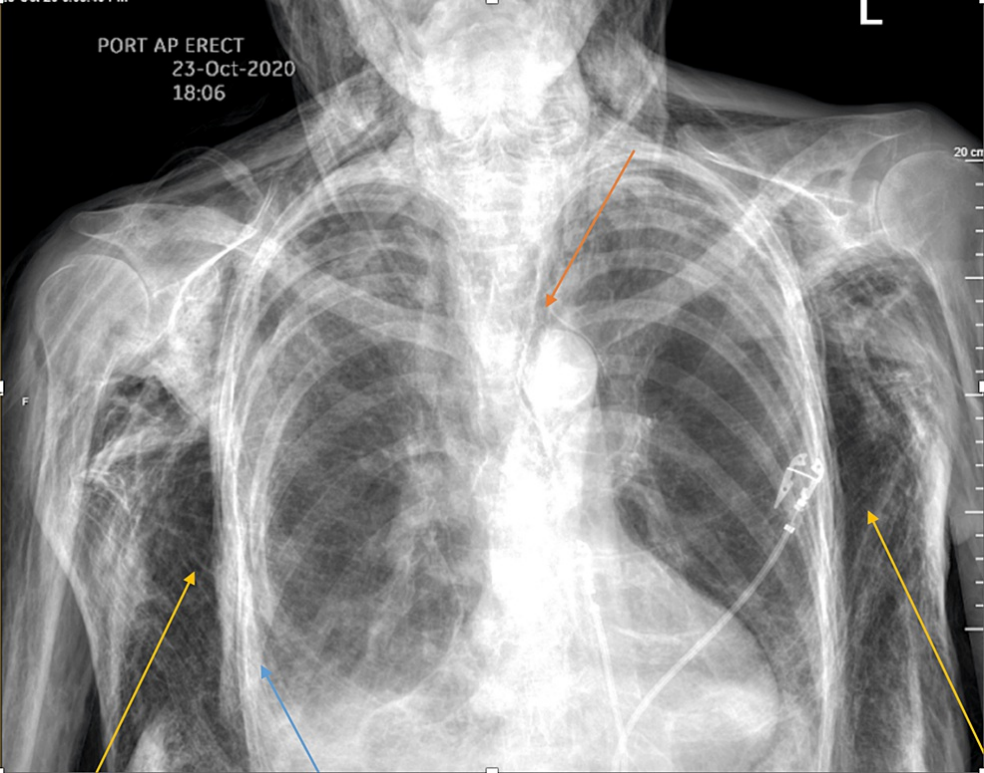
Posteroanterior upright chest X-ray. Findings notable for extensive soft-tissue subcutaneous emphysema (yellow arrows), pneumomediastinum (orange arrow), and small right hydropneumothorax (blue arrow).
